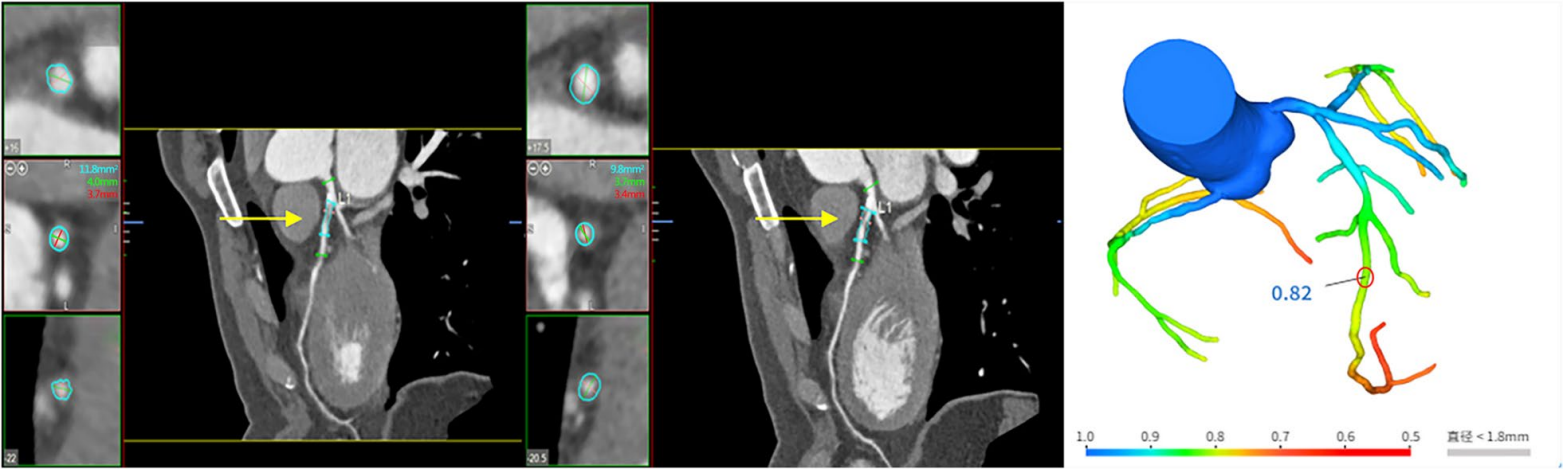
A case example of coronary stenosis on cCTA-based CDI and CT-FFR analysis. A 53-year-old man without MACEs who underwent CDI and CT-FFR analysis based on cCTA. A CDI analysis based on cCTA demonstrated that the lumen area was 11.8 mm^2^ at the early diastolic (40%) of the left anterior descending (LAD) artery 5 mm distal to the narrowest of the left main coronary artery. B CDI analysis based on cCTA demonstrated that the lumen area was 9.8 mm^2^ at the mid diastolic (75%) of the LAD artery 5 mm distal to the narrowest of the left main coronary artery. C shows no hemodynamically significant LAD lesion with a CT-FFR value of 0.82. The green data indicates the maximum diameter and red data indicates the minimum diameter. The red circle indicates that the measured position is the left anterior descending branch located distally 2 cm to the stenosis. MACEs, major adverse cardiac events; cCTA, coronary computed tomography angiography; CDI, coronary distensibility index; CT-FFR, cCTA-derived fractional flow reserve
